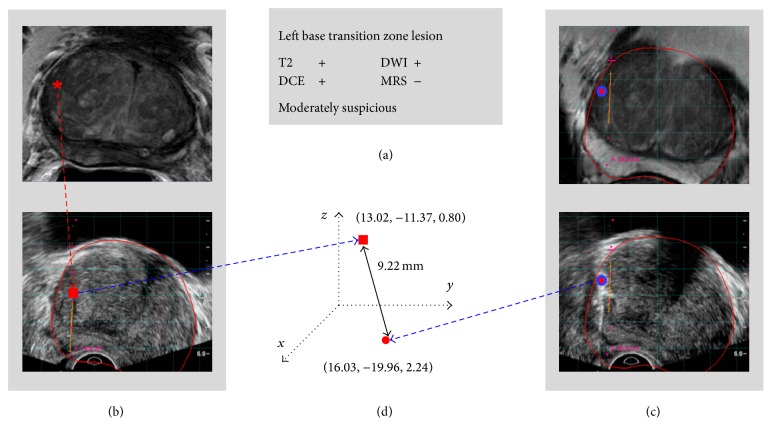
Method for calculating target location difference between visual registration and semiautomated MRI-US fusion. (a) MRI detects lesions (MRI reading). (b) A suspicious lesion is visually correlated and estimated on the US image (red rectangle). (c) The lesion is also registered through MRI-US fusion system (red circle). (d) The spatial difference between the estimated target using just ultrasound and actual target using EM-based MRI-US fusion is calculated. T2: T2-weighted, DWI: diffusion-weighted, DCE: dynamic contrast-enhanced, and MRS: MR spectroscopy.
